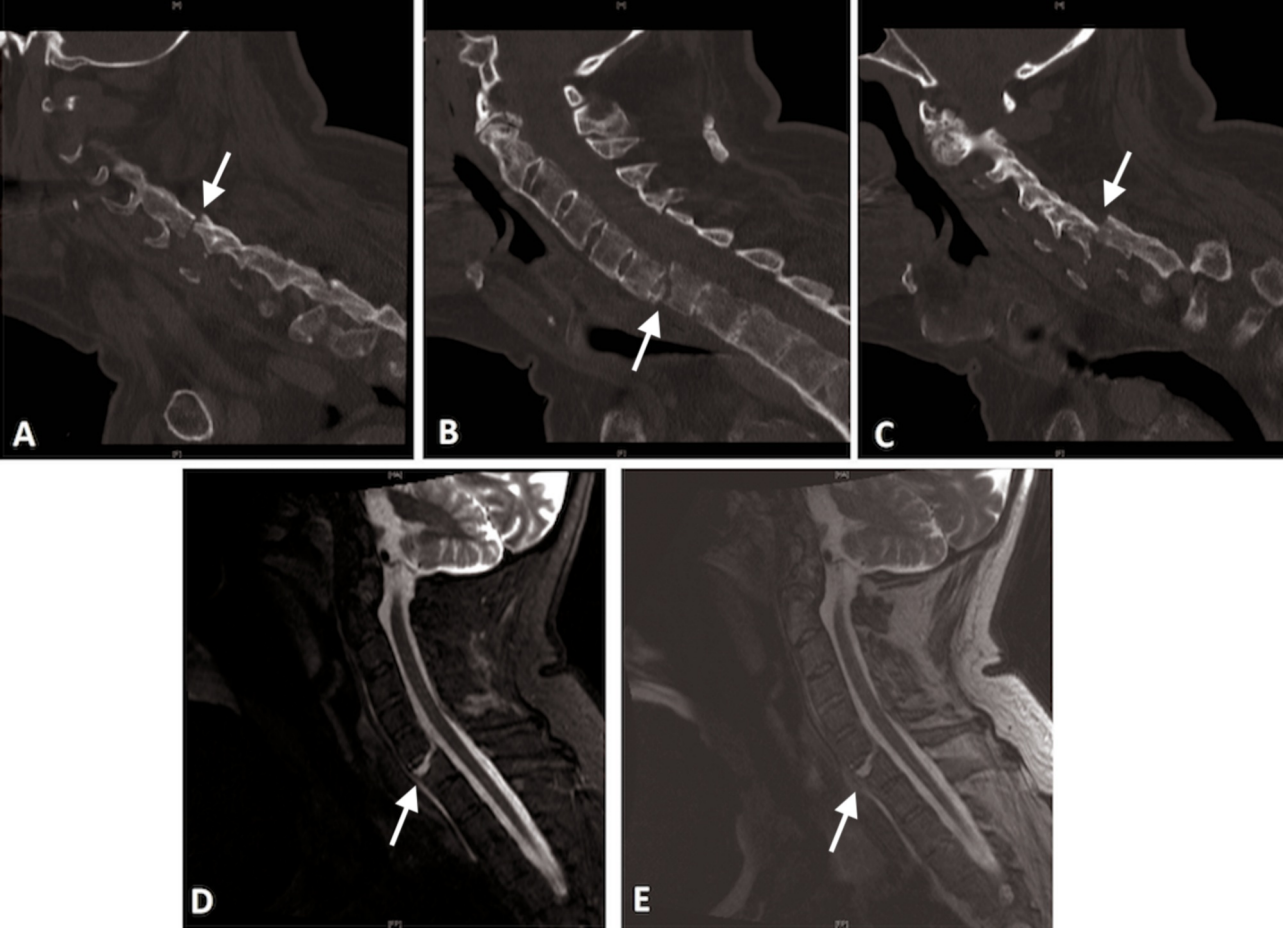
Preoperative CT scan of cervical spine CT scan of the cervical spine demonstrating a three-column extension fracture at C6/7 in the setting of ankylosis of the levels above and below. Note the three-column involvement with fracture through the ossified disk (B) and involvement and displacement of the facets (A and C). Short tau inversion recovery (D) and T2-weighted (E) sequences demonstrating increased signal segmentally at the level of the fracture indicate total segmental disruption.
